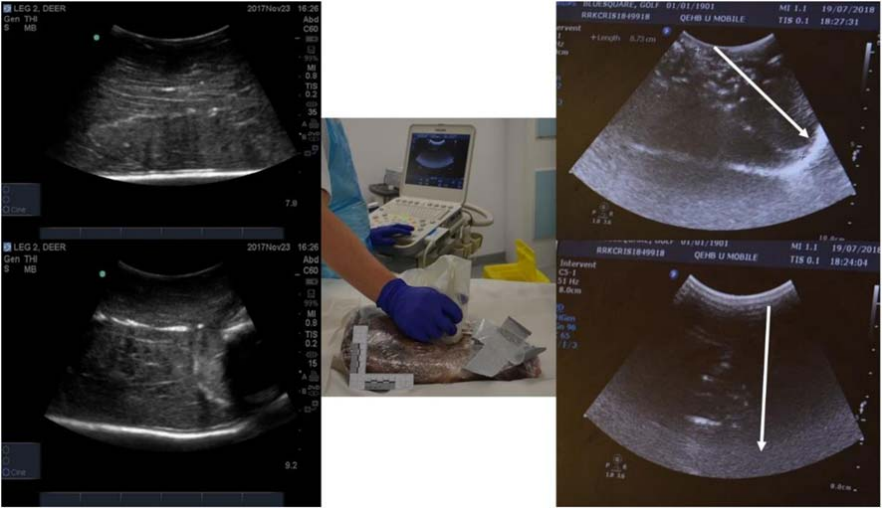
Left, top, and bottom—pre-contrast, pre-shoot ultrasound images; centre—ultrasound in progress, demonstrating curvilinear probe compression of limb soft tissue; right, top, and bottom—post contrast injection ultrasound, highlighted areas represent GSW track, arrows indicate projectile direction of travel
